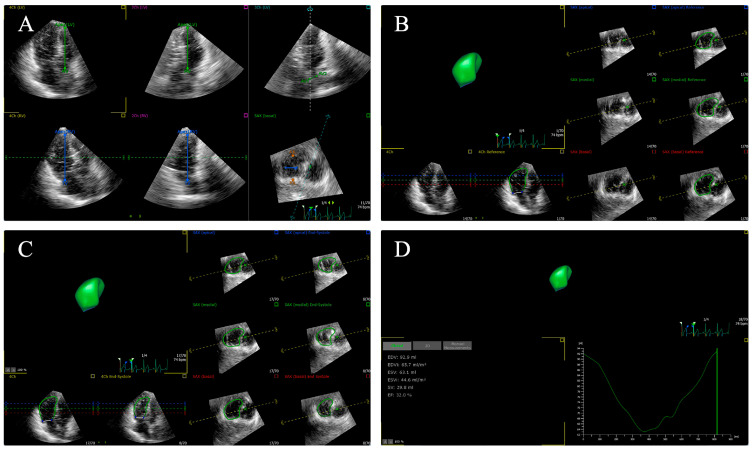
Longitudinal strain of right ventricular free wall and septum using three-dimensional speckle tracking echocardiography. (A) Setting reference points; (B,C) right ventricular endocardial border tracking; (D) longitudinal strain of right ventricular free wall and septum are automatically generated.
